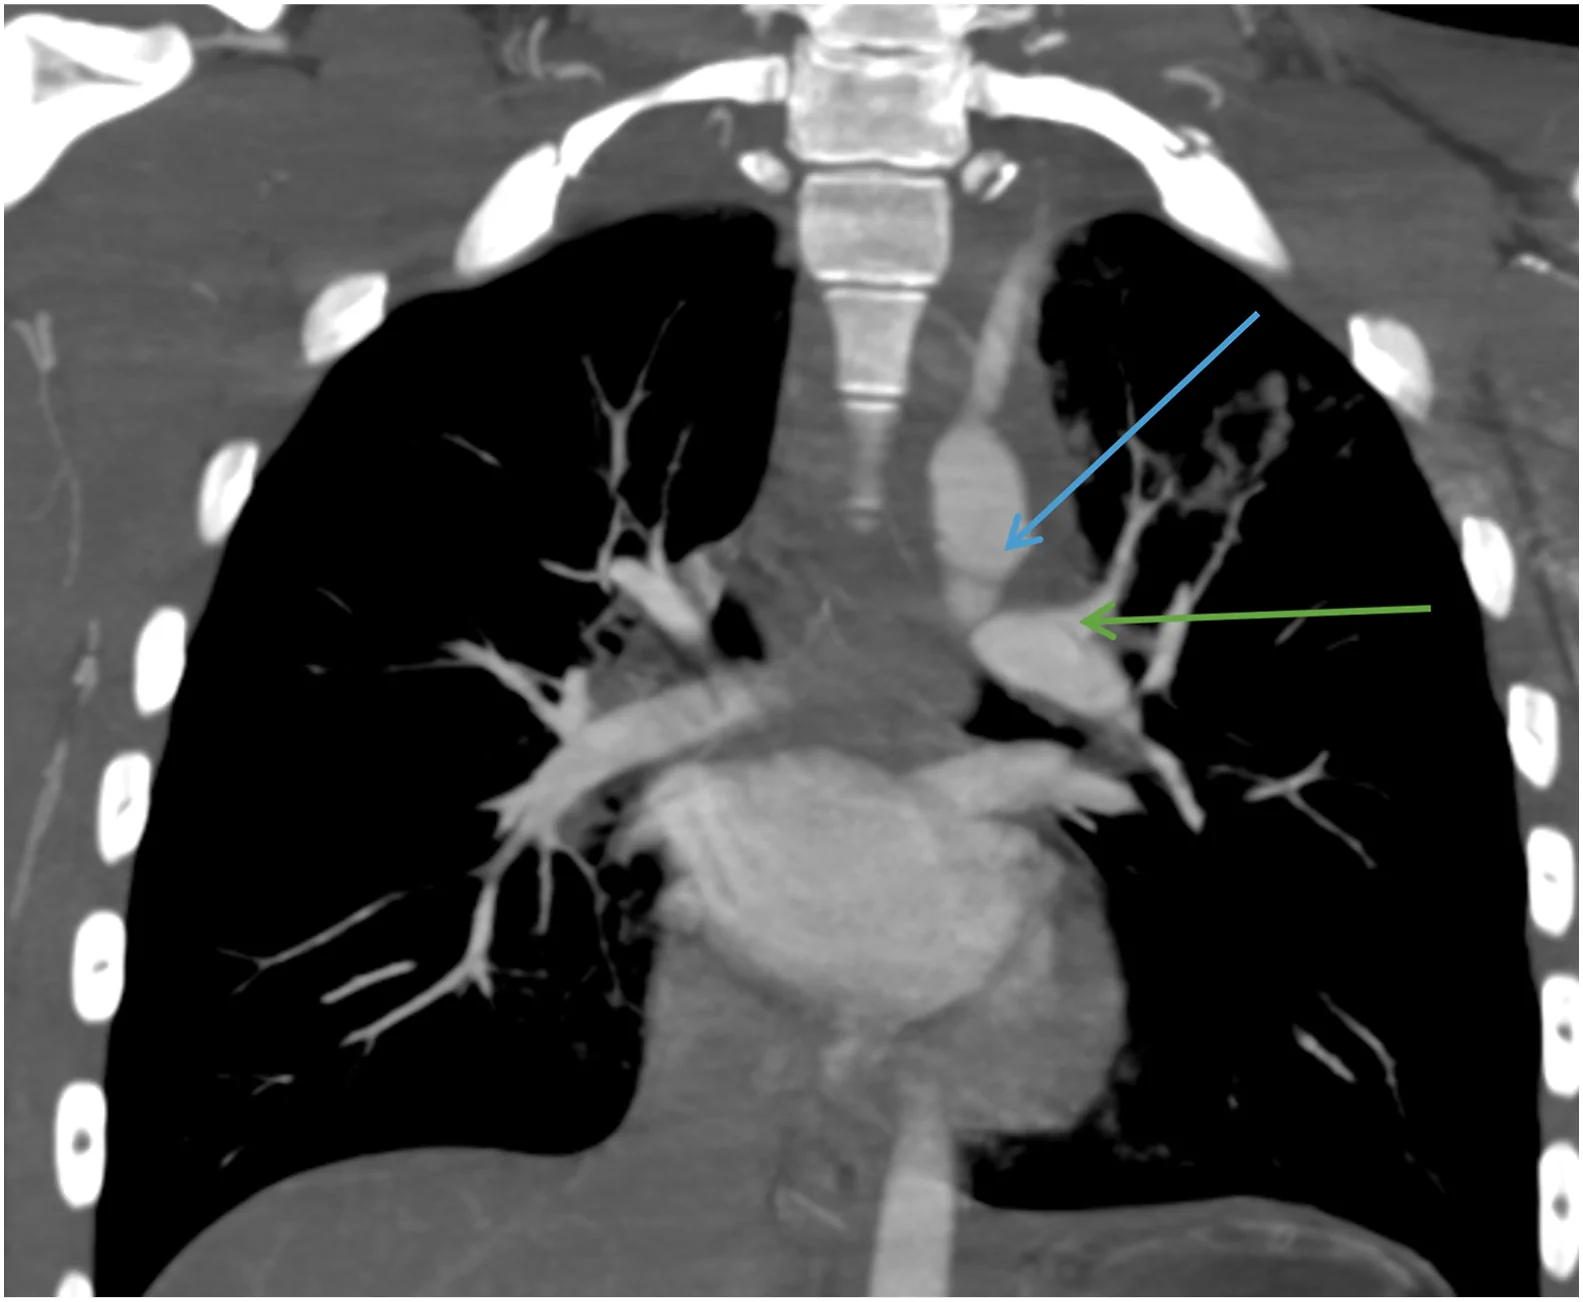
Coronal view chest computed tomography of the donor with the blue arrow pointing to the aortic injury (so called, “distracting injury”) and the green arrow pointing to the left pulmonary artery injury (“missed injury”). The imaging demonstrated an aortic injury in the vicinity of the ligamentum arteriosum.
